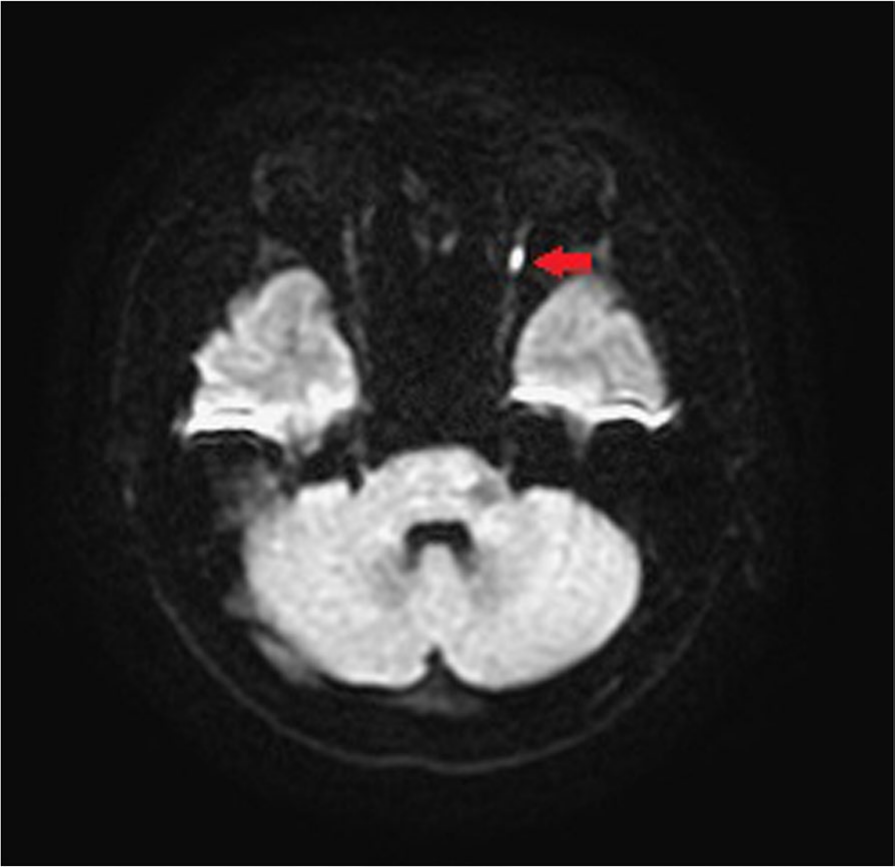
T2WI DWI showed focal high intensity signal at the left optic nerve. (Red arrow)
